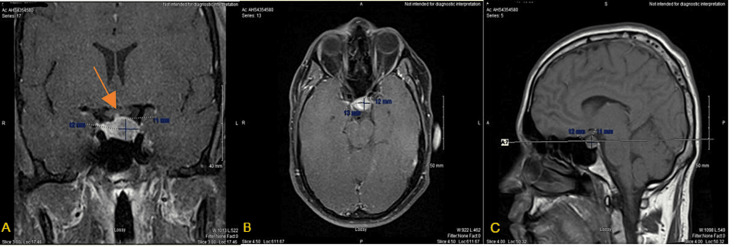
Initial MRI of a sellar mass (A) Axial T1, (B) coronal T2, and (C) sagittal T1 displaying sellar mass measuring 12 x 13 x 11 mm (L x W x H). You can observe a slight visualization of the optic chiasm (arrow in (A)).
